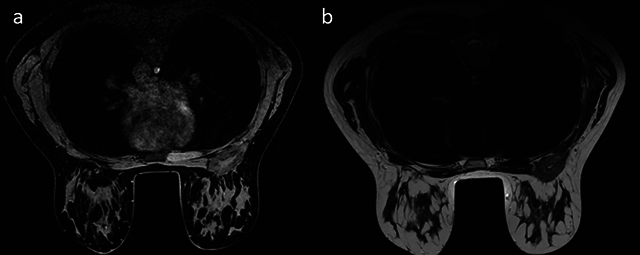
a. MRI T1-weighted Dixon water image. b. T2-weighted image. Deep right upper mammary lesion, at least partially intra-pectoral, oblong in shape, with irregular margin.
